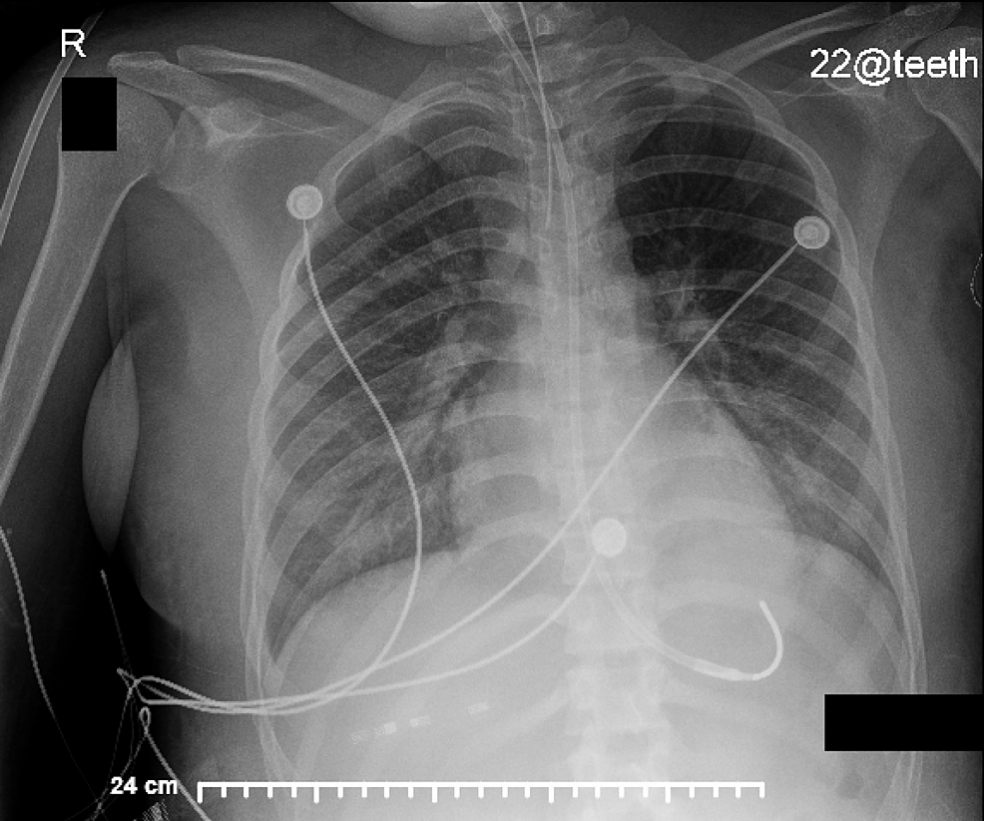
The chest X-ray shows decreased pulmonary edema.
